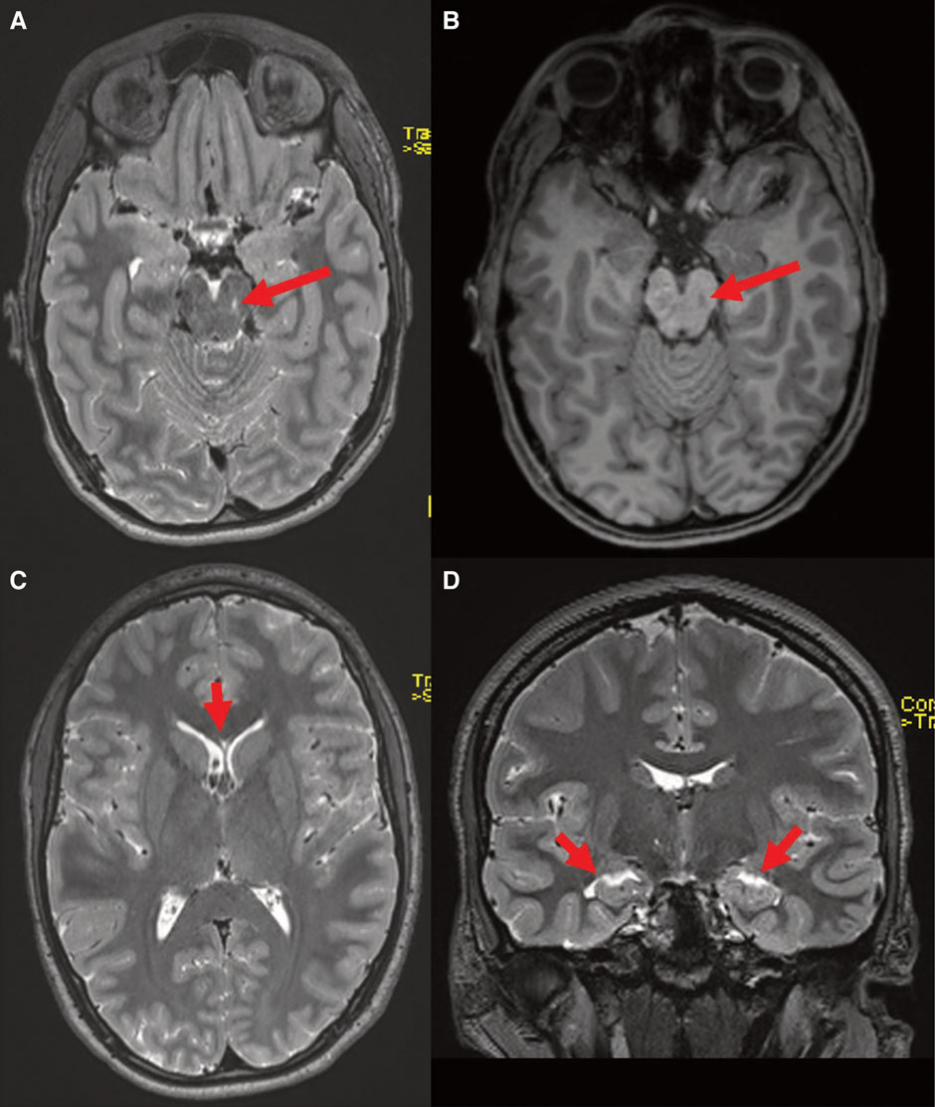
Brain MRI. Brain MRI showing a left midbrain lesion: an axial 2-mm reformation of the 3D T2 sequence (A) with a faint crescenteric focus of T2 signal hyperintensity (red oblique arrow). This correlates with the T1 signal hypointensity (red oblique arrow) of the 3D T1 GRE sequence (B). Further, an axial 2-mm reformation of the 3D T2 sequence (C) revealed the presence of a cavum septum pellucidum (total length, 14 mm; vertical red arrow). The coronal 2-mm reformation of the 3D T2 sequence (D) shows subtle widening of the temporal horns of the lateral ventricles bilaterally and a slight reduction in volume of the hippocampi (red oblique arrows). Brain MRI was performed using the following sequences: 3D T1 gradient echo (GRE); 3D diffusion tensor imaging (DTI); 3D T2 turbo spin echo (TSE); 3D fluid-attenuated inversion recovery (FLAIR); coronal T2-weighted (2-mm slice thickness); axial T2*; and SWI. Images were assessed using a structured report based on the NINDS Common Data Elements (CDEs) for traumatic brain injury. Automated volumetric segmentation was performed using the 3D T1 GRE sequence and the software-tool md.brain v1.1.1, which compares individual values to a normative database comprised of several thousand persons 18–92 years of age while accounting for age, sex, and intracranial volume. 3D, three-dimensional; MRI, magnetic resonance imaging; NINDS, National Institute of Neurological Disorders and Stroke; SWI, susceptibility weighted imaging.
